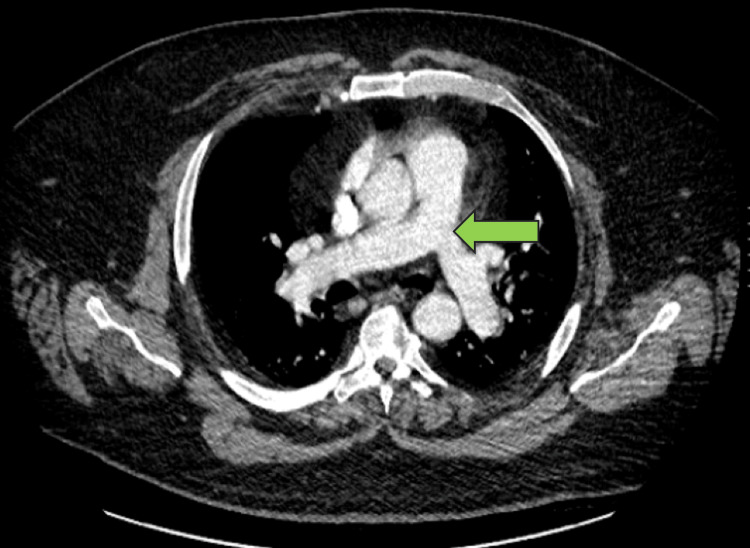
CT chest pulmonary embolism protocol on the day of admission, demonstrating patent pulmonary arteries as shown by the green arrow.
